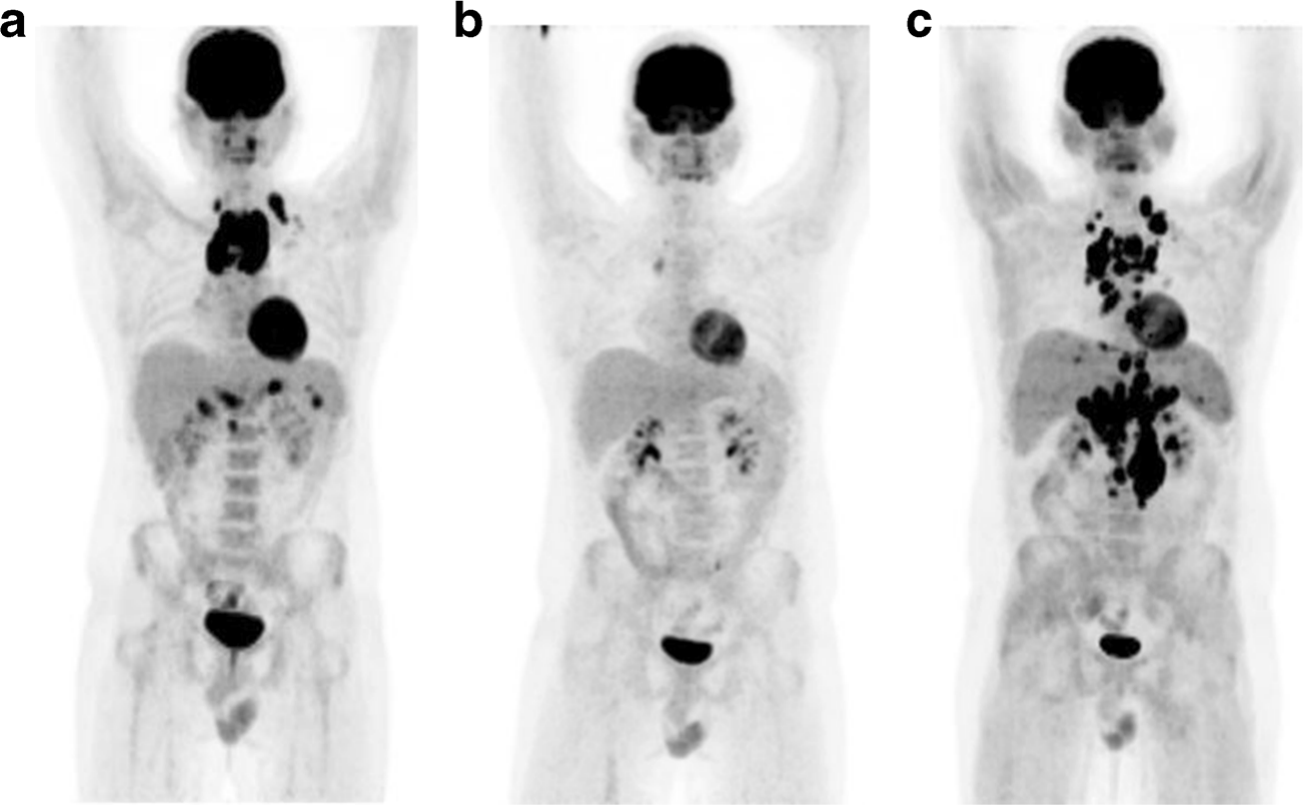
A 15-year-old boy with Hodgkin lymphoma. Maximum intensity projections of FDG PET. a The initial staging PET image reveals stage III disease. b The interim PET image after two cycles of chemotherapy (OEPA) shows PET-positive residual uptake at the right side of the mediastinum (Deauville score 4). c The PET image 4 months later shows extensive relapse of the disease (courtesy of Prof. Reinhardt, Pius Hospital Oldenburg)
